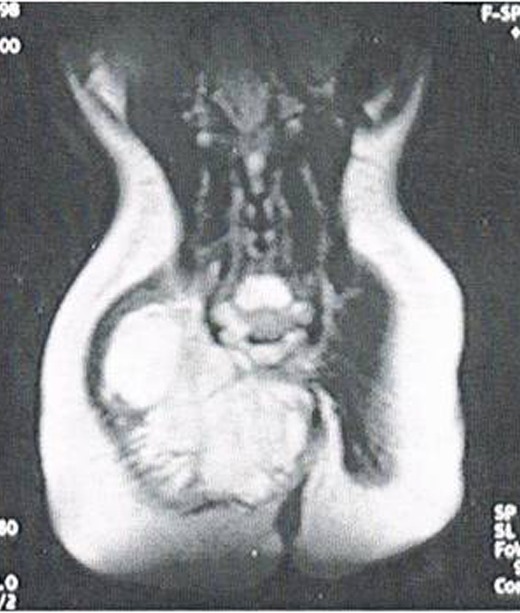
Figure 1: Abdominal and pelvic magnetic resonance imaging of the pelvis displays a large heterogeneous pelvic mass extending into the right gluteal region with significant mass-effect on adjacent organs. It shows infiltration of lower sacral spinal canal.
